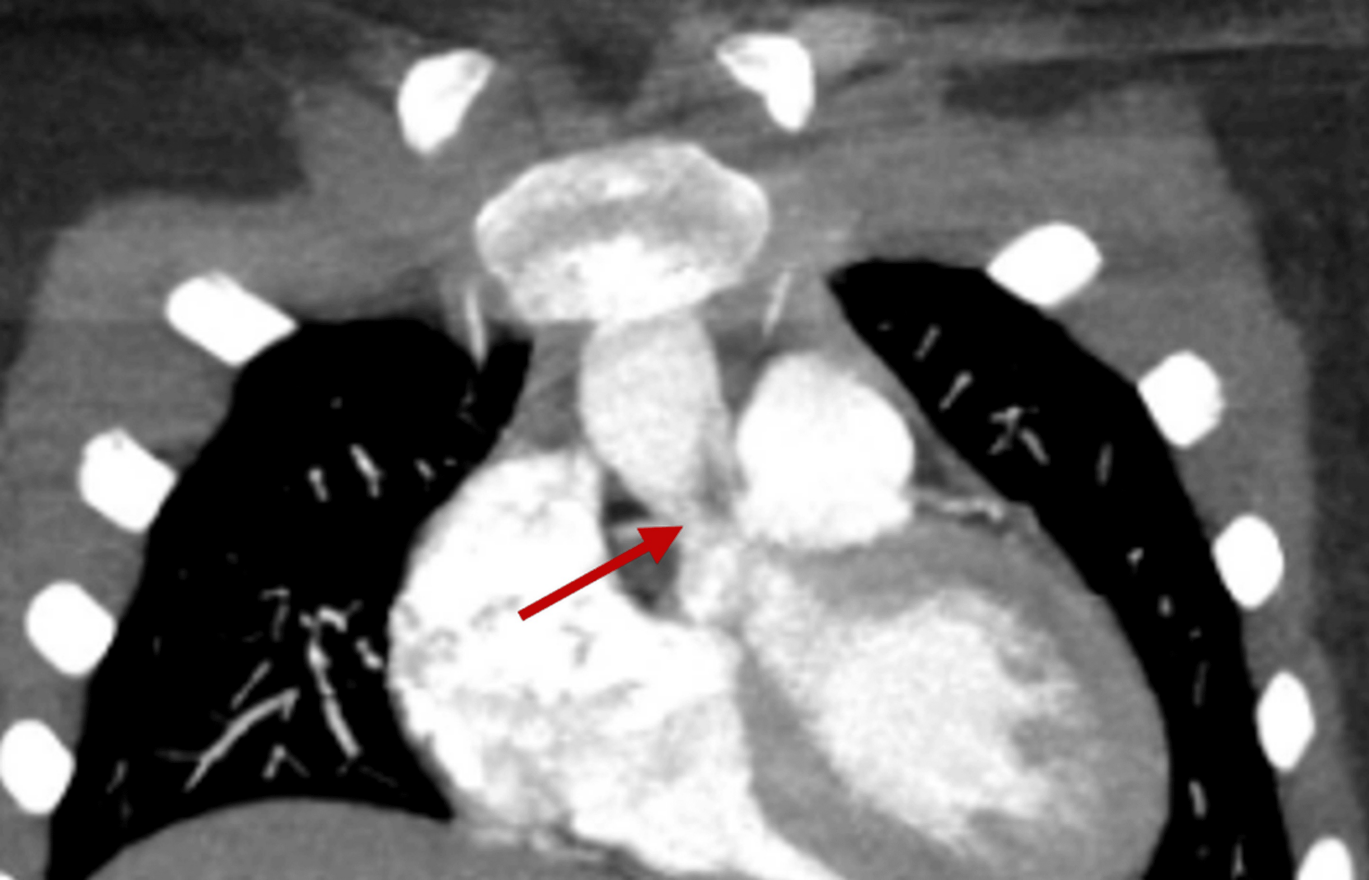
Chest CT (coronal view) showing a hypodense lesion (red arrow) at the level of the noncoronary cusp, extending to the aortic valve junction. Findings are suspicious for a peri-annular abscess
